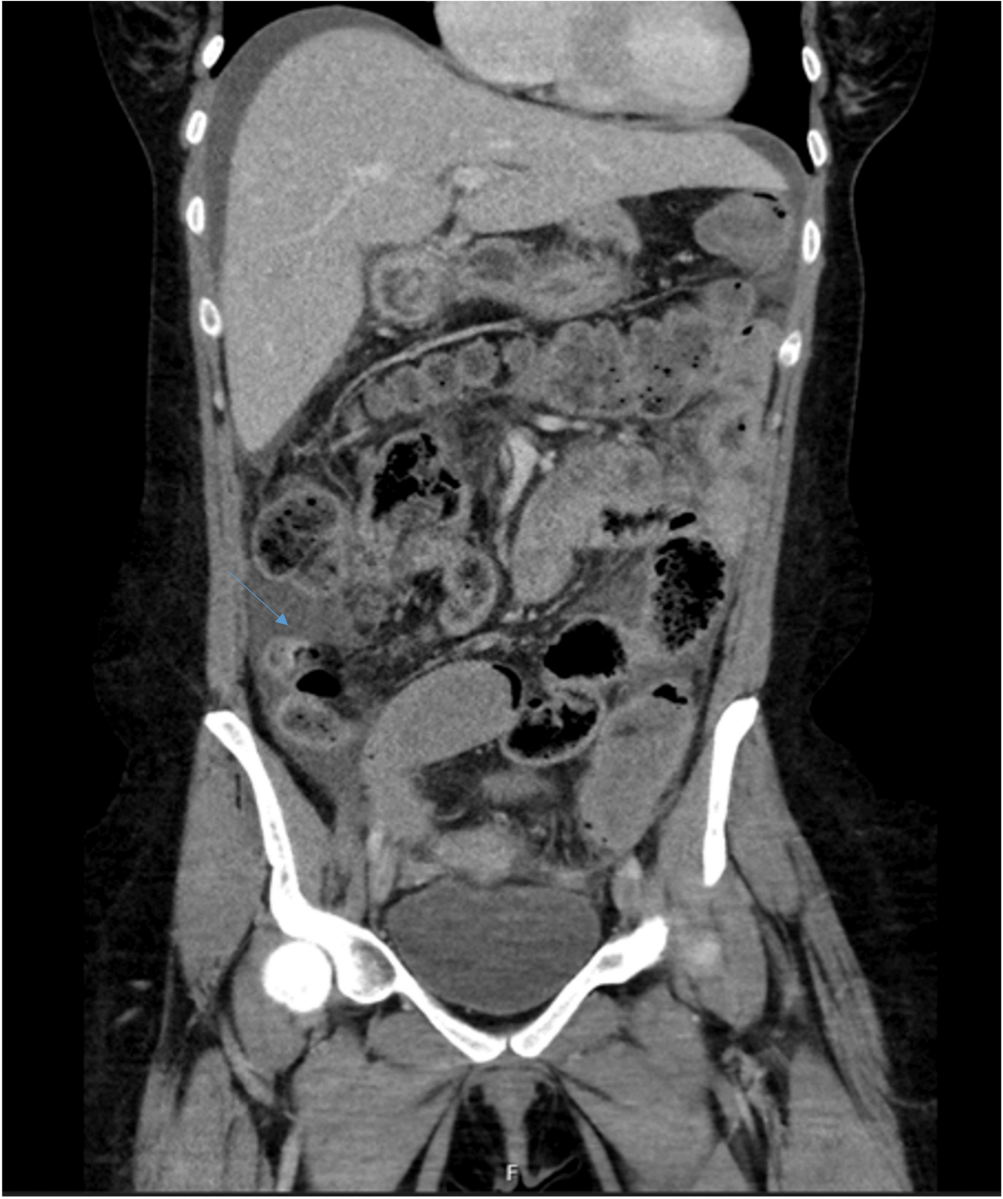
Coronal contrast-enhanced abdominal CT showing free intraperitoneal fluid (arrow), suggestive of a peritonitis (without pneumoperitoneum) in a patient with prior Roux-en-Y gastric bypass (RYGB).
